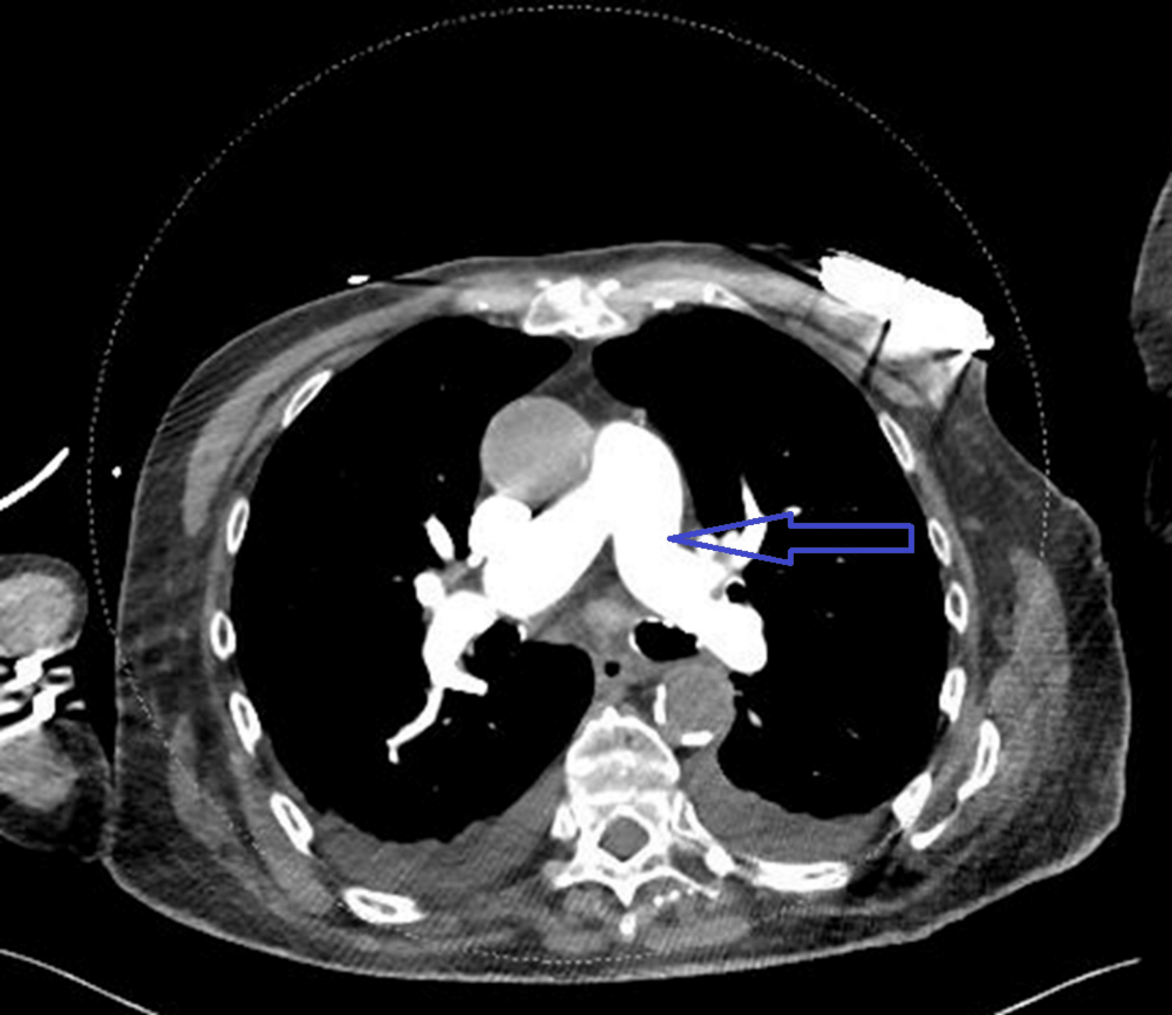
Computed tomography angiography of the chest showing absence of pulmonary embolism.
